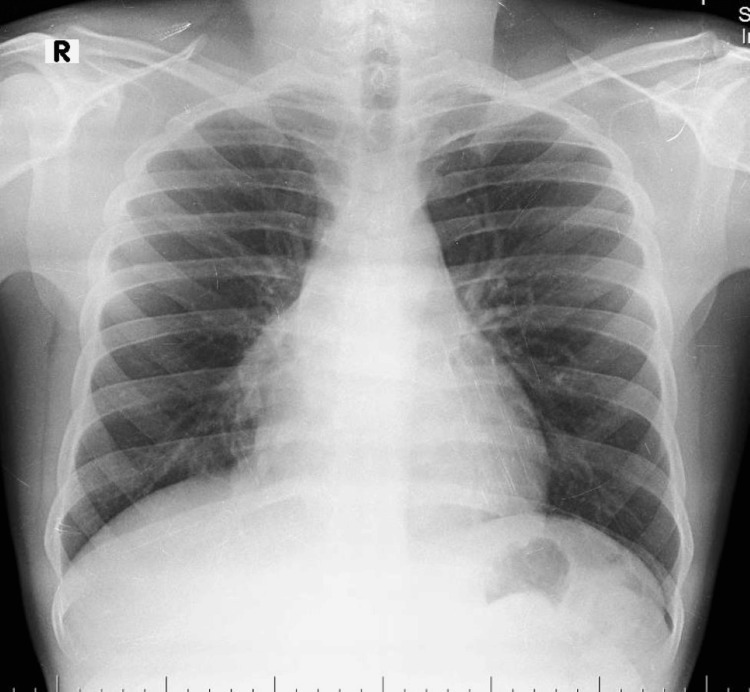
Chest radiograph following therapeutic thoracentesis and steroid therapy, a comparative reduction in the size of the mediastinal mass and resolution of the pleural effusion were noted.
